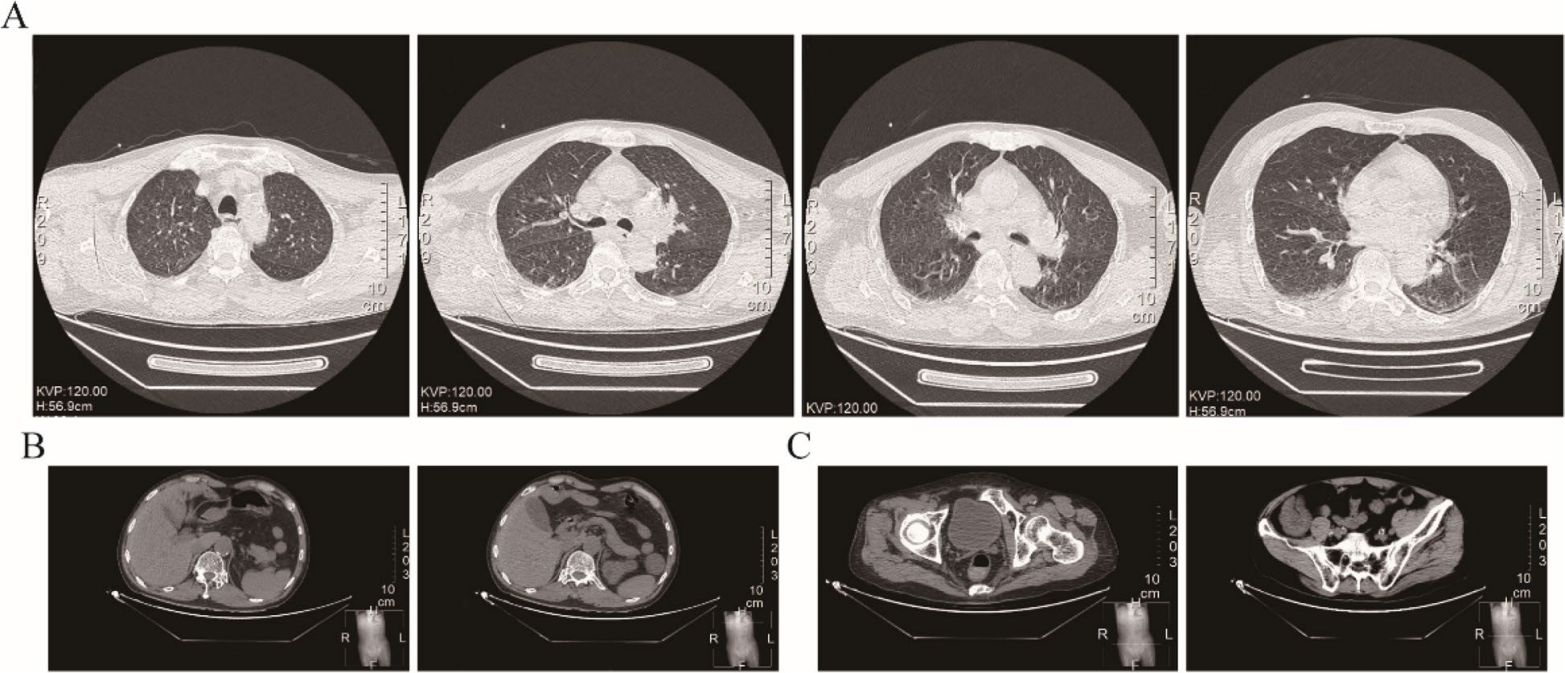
Chest and abdomen/pelvis CT: a small amount of pneumonia in both lower lung, hepatic cyst, renal cyst, prostatic hyperplasia
